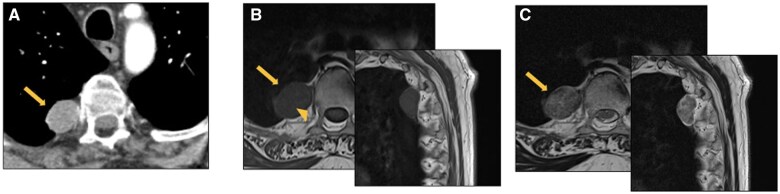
Schwannoma. (A) Contrast-enhanced CT axial image (arterial phase) shows a well-defined rounded soft-tissue lesion (arrow) in the right paravertebral mediastinum. This mass abuts the adjacent spinal nerve (arrowhead), appears hypointense on T1-weighted MR images (B) and moderately hyperintense with central hypointense foci on T2-weighted images (C), representing thickened fascicles (fascicular sign).
